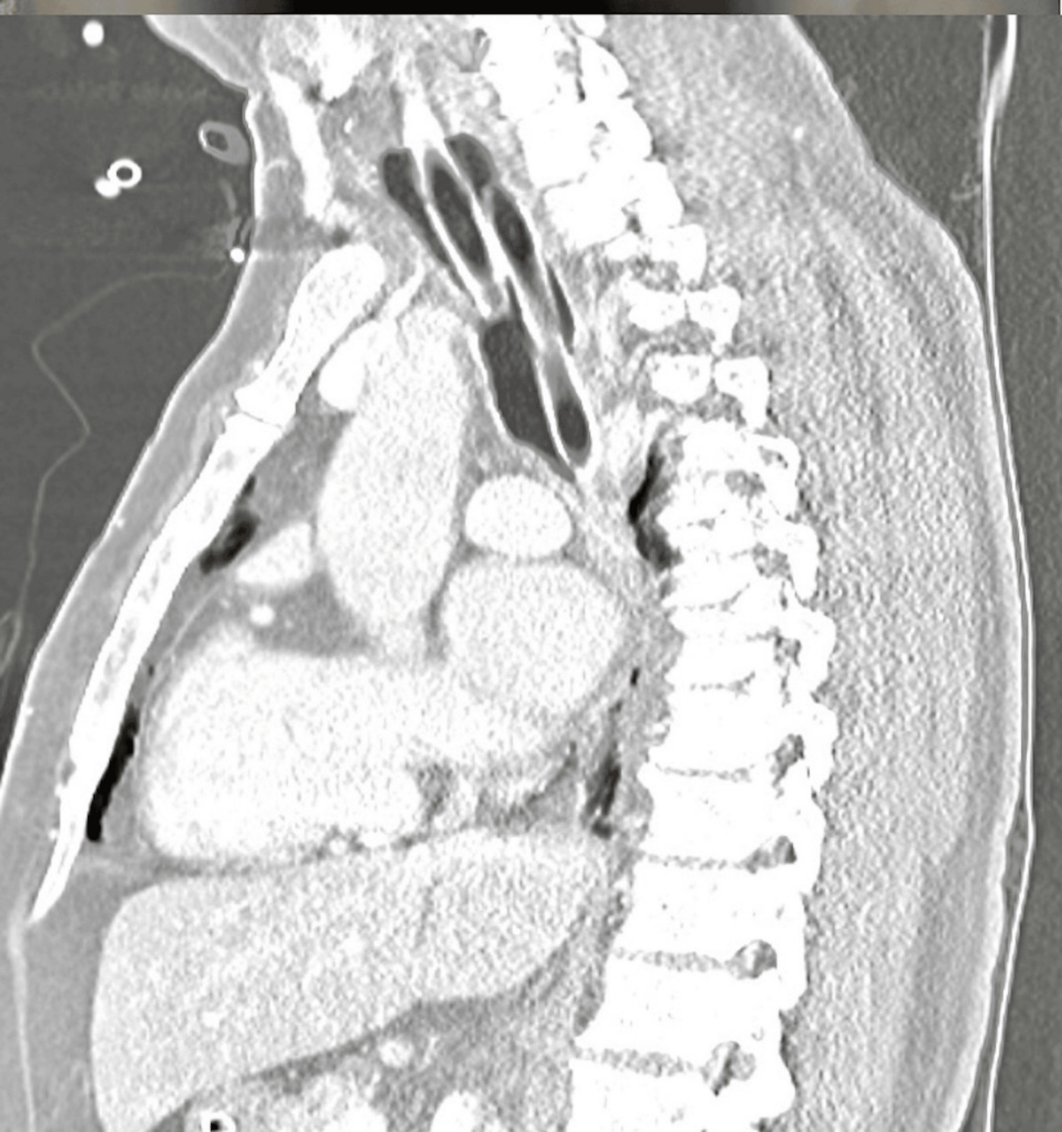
Chest CT showing nasopharyngeal airway in the lateral view.
